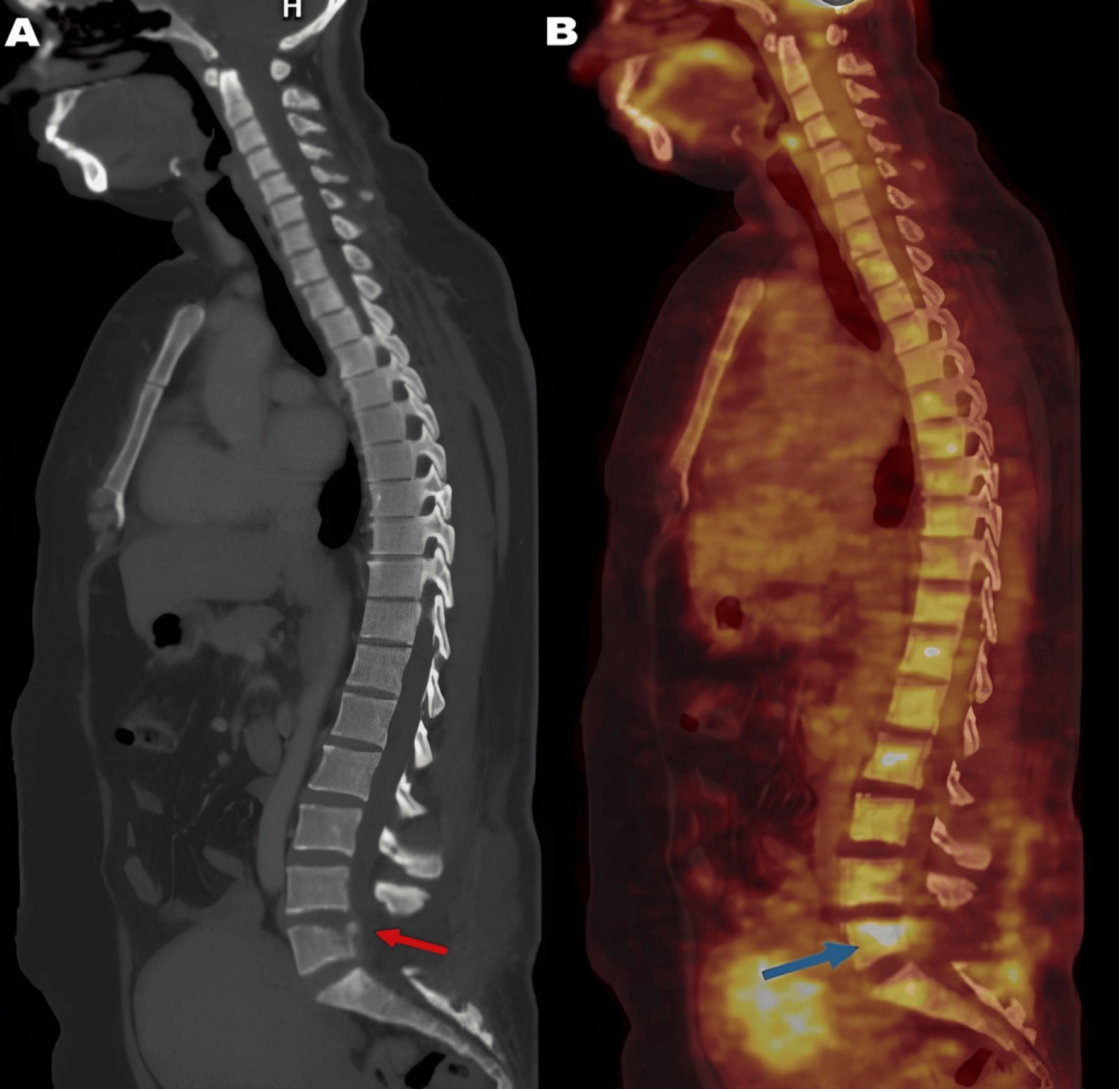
(A) Sagittal CT reconstruction of the lumbosacral spine demonstrating subtle cortical irregularity with minimal lytic erosion of the superior endplate of the L5 vertebral body. (B) Sagittal FDG PET-CT fusion image showing focal avid FDG uptake at the same site, with no additional hypermetabolic lesions.
